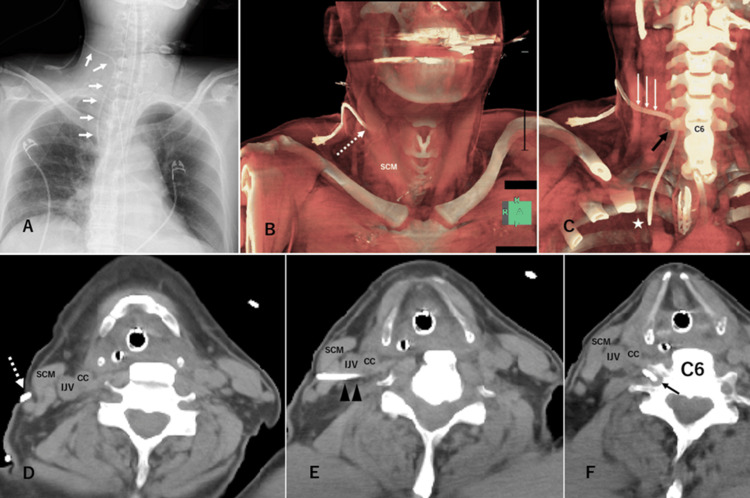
Radiological findings after the insertion of a central venous catheter (A) X-ray image and (B, C: three-dimensional-reconstructed images; D-F: axial images) computed tomography images after the insertion of a central venous catheter. The central venous catheter (CVC; white arrows in A and C) was inserted from the posterior limb of the right sternocleidomastoid muscle (SCM) (dotted arrow in D), dorsally passing (black arrowheads) the right internal jugular vein (IJV) and common carotid artery (CC) and entering the transverse foramen of C6 (black arrow in C and F). The CVC tip (white star in C) is positioned in the right brachiocephalic vein.
